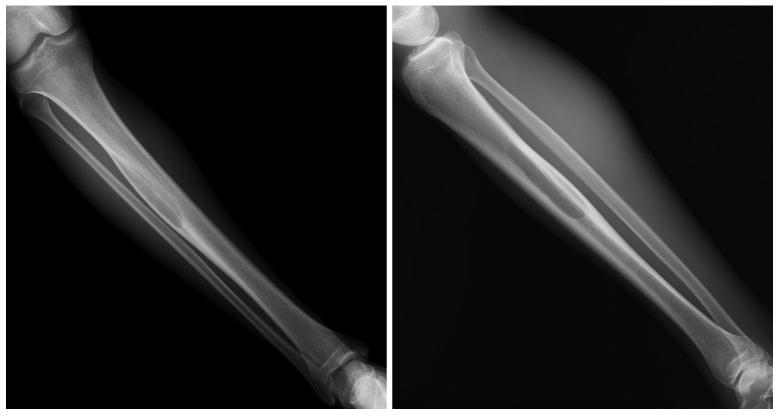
Anteroposterior and lateral radiographs showing a defined osteolytic lesion in the tibial diaphysis with mild bulging on the posterolateral side.
